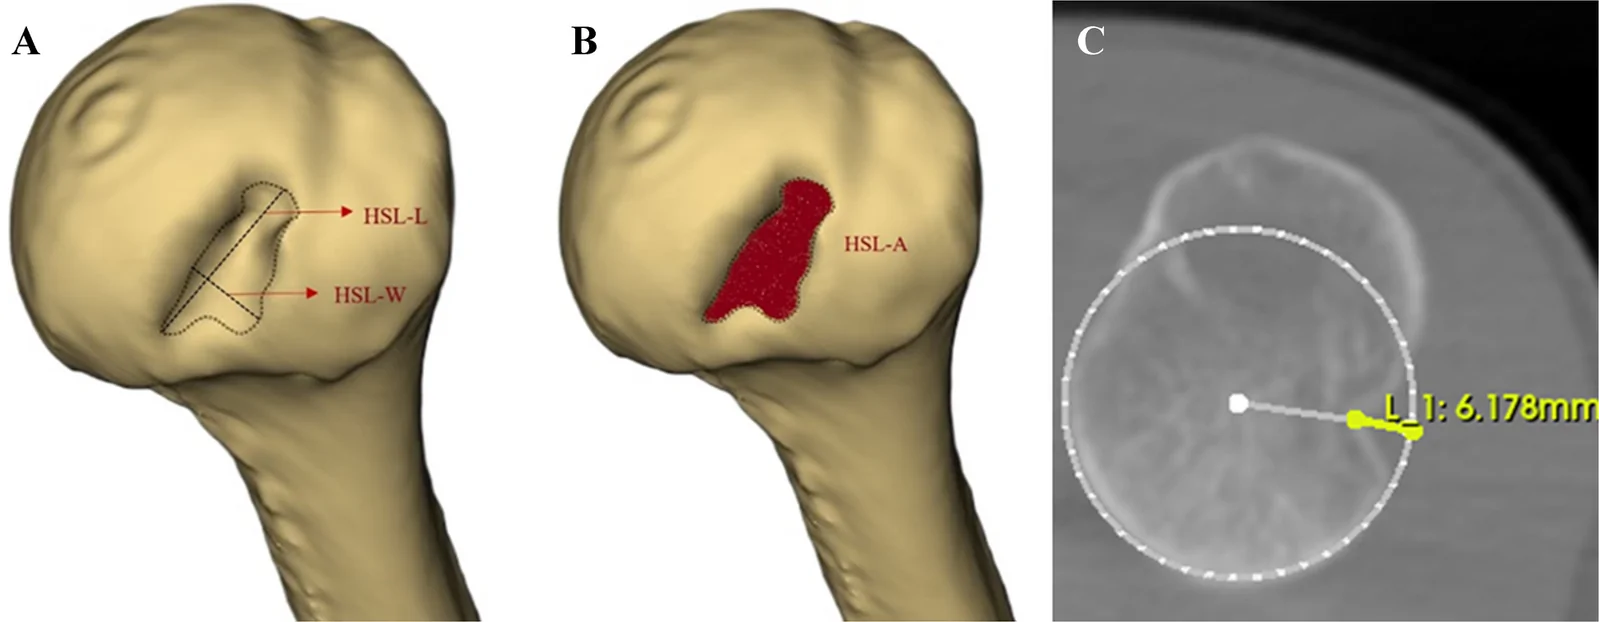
Representation of Hill-Sachs lesion (HSL)-related measurements on 3D-CT. A HSL length (HSL-L) and HSL width (HSL-W) measurement. B HSL area (HSL-A) measurement. C HSL depth (HSL-D) measurement
